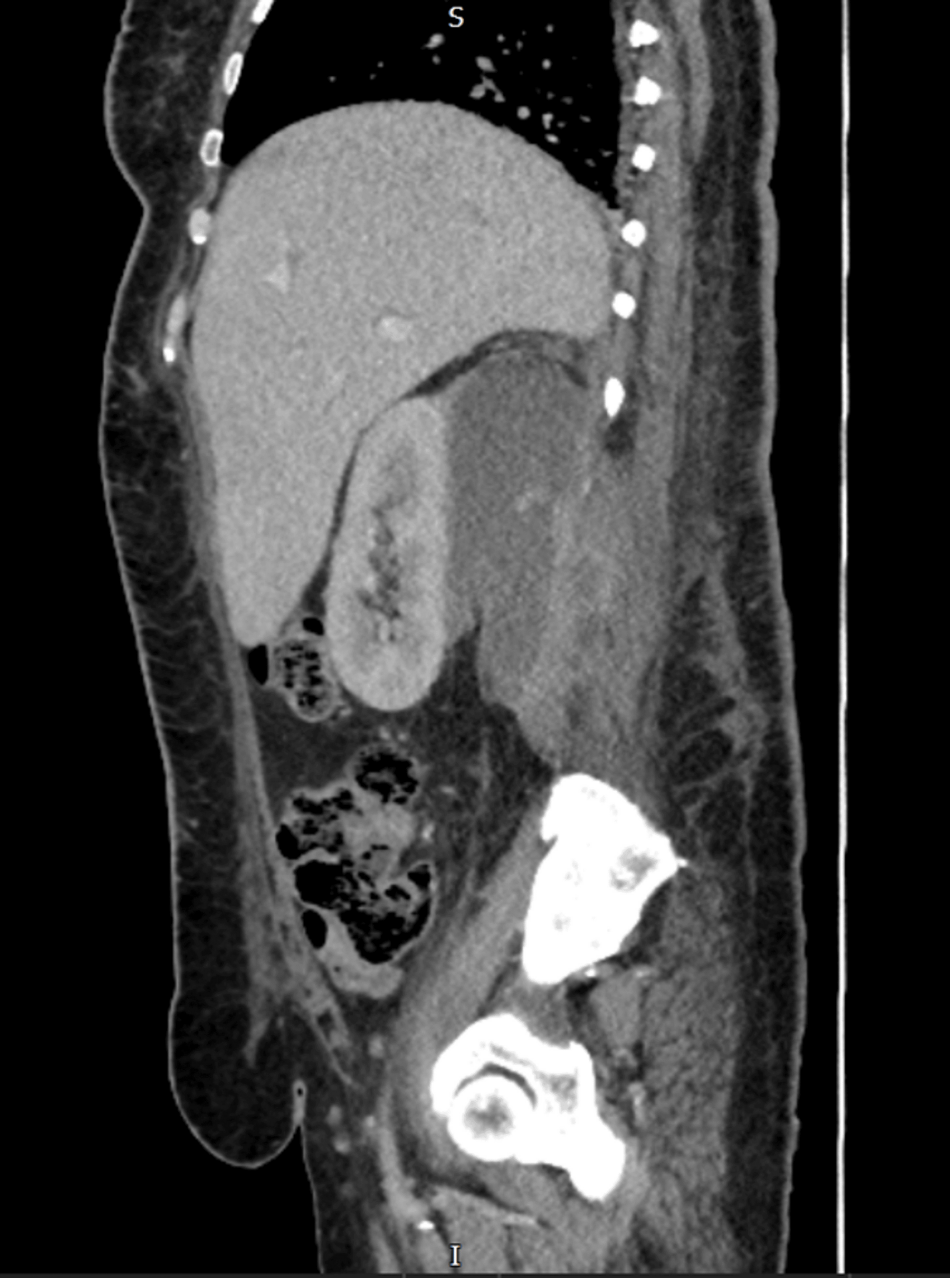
CT abdomen pelvis with contrast sagittal view done on day 3 shows right posterior perinephric collection measuring 7.7 cm x 5.3 cm, extending along the entire length of the kidney, and involving both the right psoas and right erector spinae muscles CT: computed tomography
